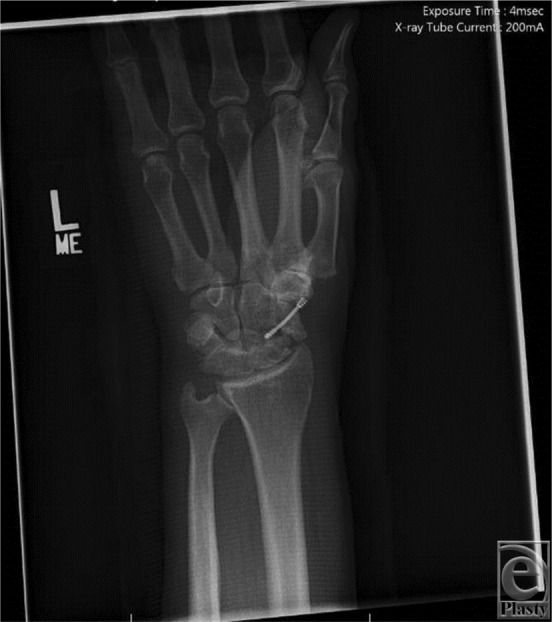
Preoperative posteroanterior radiograph of transscaphoid transcapitate perilunate fracture-dislocation demonstrating previous scaphoid open reduction internal fixation resulting in nonunion and scaphoid nonunion advanced collapse wrist. Also demonstrated are loss of carpal height and alternation of Gilula's lines.
